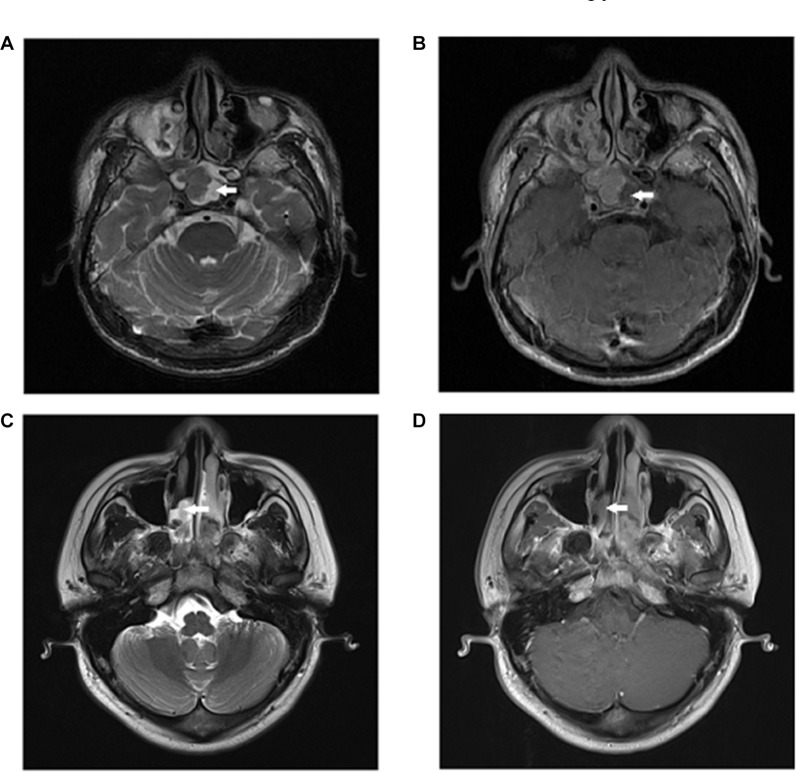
Primary tumor inflammation in two NPC patients (A) Axial T2-weighted and (B) contrast-enhanced T1-weighted MR images of a 41-year-old man show primary tumor inflammation in the sphenoid sinus; (C) Axial T2-weighted and (D) contrast-enhanced T1-weighted MR images of a 29-year-old man show primary tumor inflammation in the nasal cavity.
